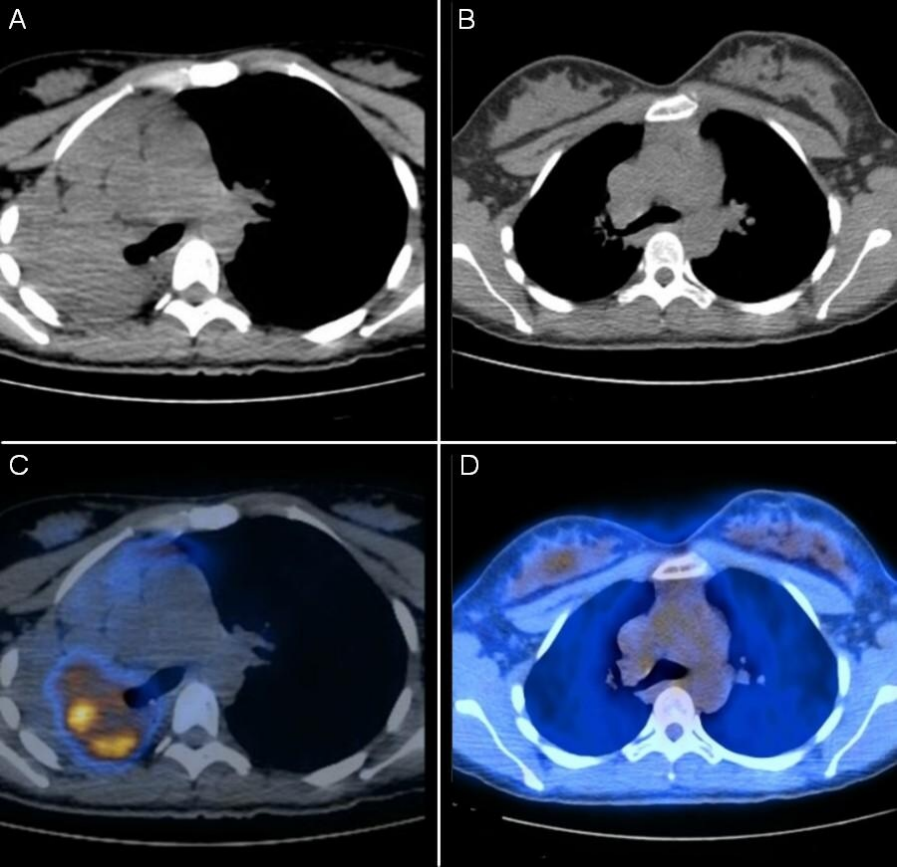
Pre-treatment and post-treatment images Pre-treatment CT chest (A) and fused PET/CT image (C) show large right hilar mass that obstructs the right main bronchus resulting in collapse of the right lung and mediastinal shift to the right. Post-treatment CT Chest (B) and fused PET/CT image (D) show complete radiographic response after treatment with chemotherapy.
